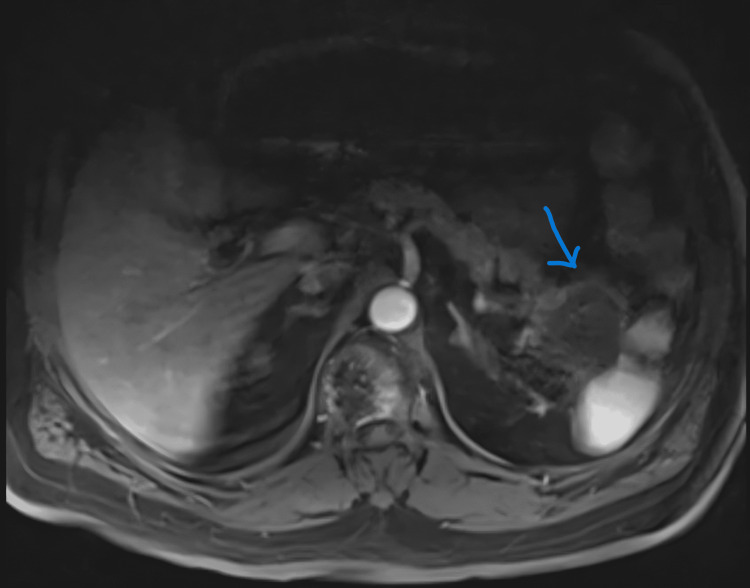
MRI of the abdomen demonstrating a 5.4 cm pancreatic tail mass with splenic invasion Arrows point to the pancreatic lesion and its interface with the spleen. MRI: magnetic resonance imaging
